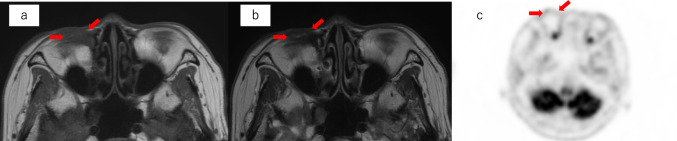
MRI and PET images 4 years after radiation therapy. The red arrows show a smaller tumor. a T1-weighted MRI. b T2-weighted MRI. c PET no longer shows FDG accumulation
